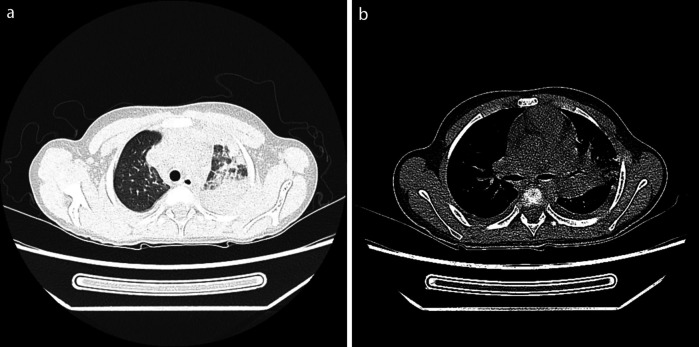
Chest CT. Left lobar pneumonia (a) and pleural effusion (b).
